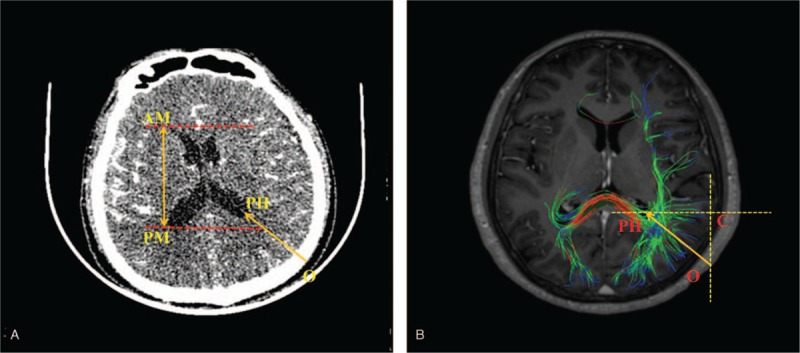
(A) The data and the puncture point from the occipital lobe in computed tomography image. (B) The puncture point and angle in diffusion tensor image.
